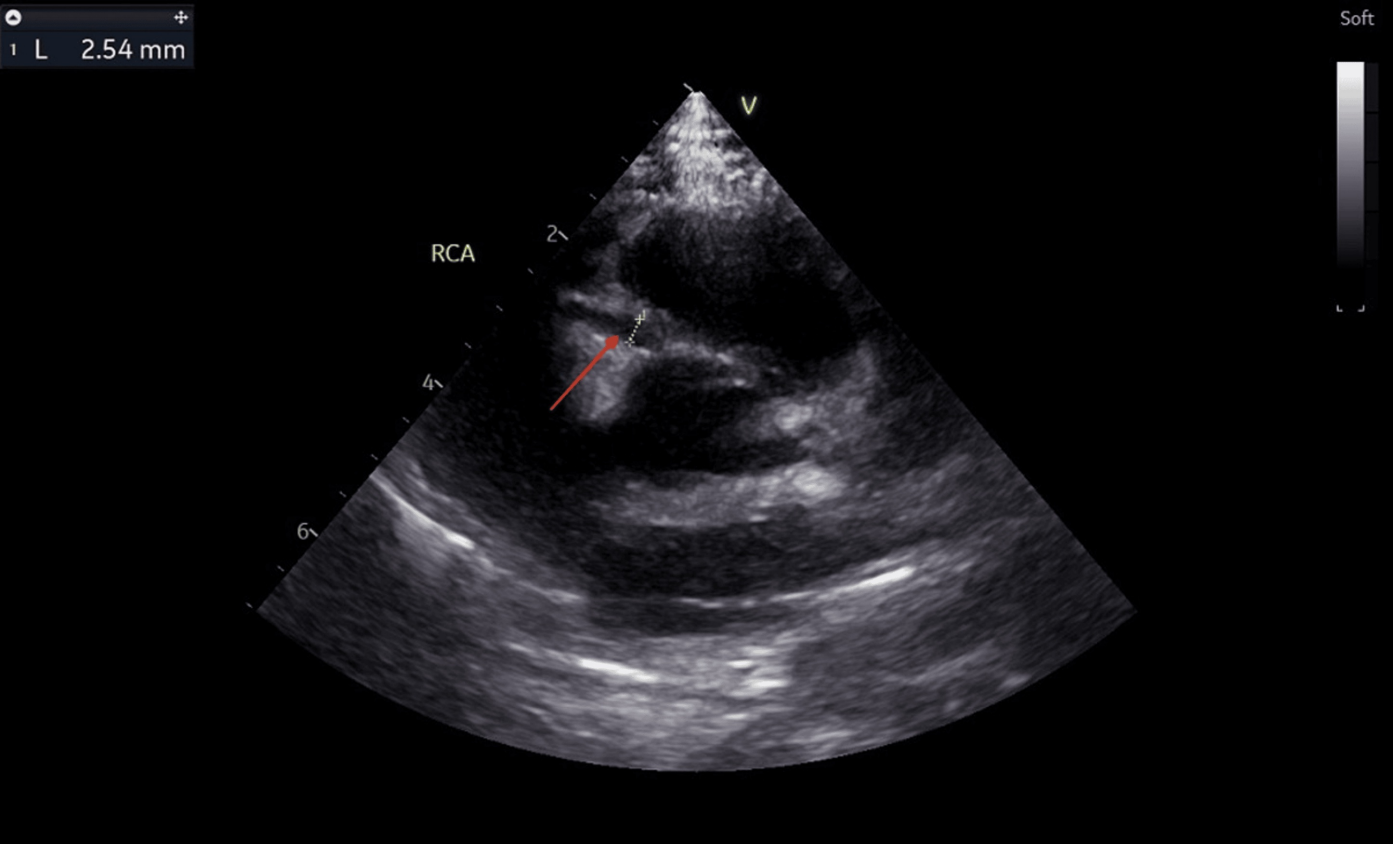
Echocardiogram showing a dilated right coronary artery. The red arrow points at the dilated right coronary artery measuring 2.5 mm (Z score: +2.83).
